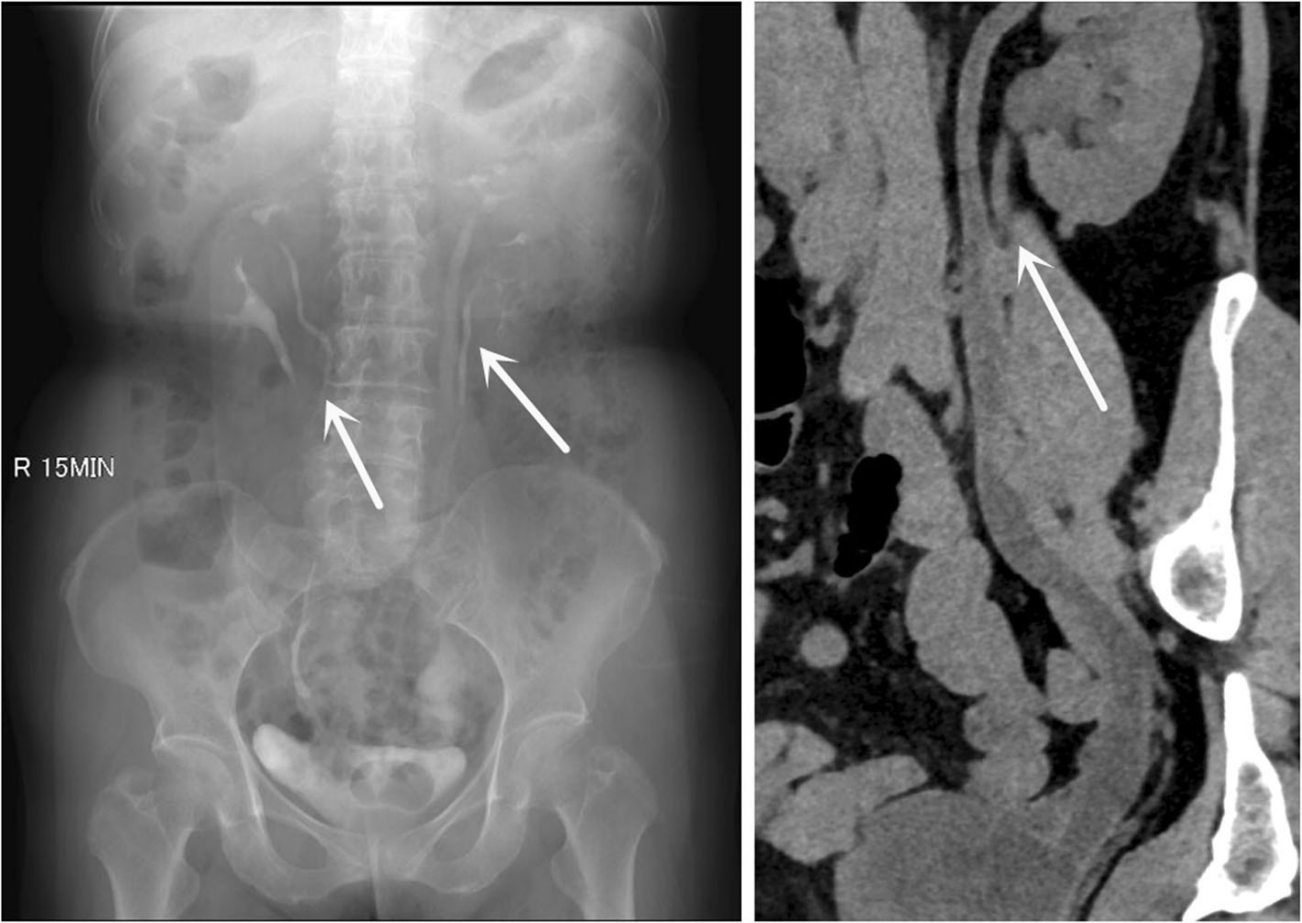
Intravenous pyelogram and CT scan revealed bilateral incomplete duplex kidney and ureter
